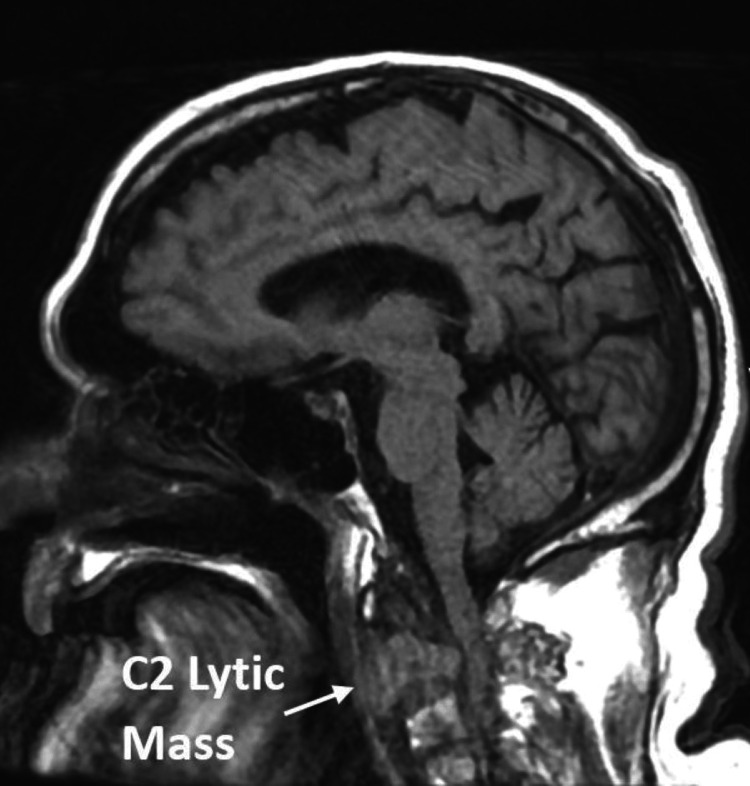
MRI of the cervical spine exhibiting the expansile marrow-displacing, enhancing process involving the C2 and odontoid process.
